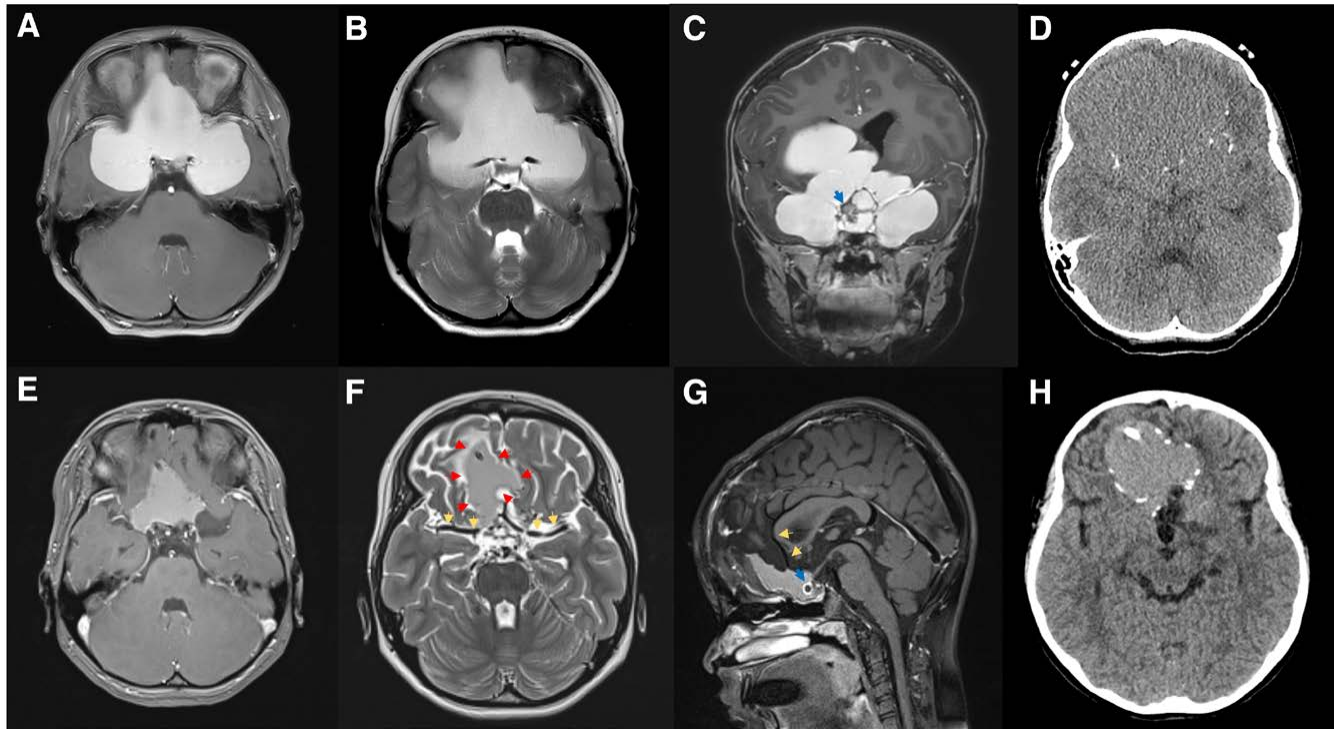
The radiologic findings of cystic craniopharyngioma following long-term cystic aspirations via the Ommaya reservoir. Preoperative brain magnetic resonance imaging (MRI) revealed a 10 cm-sized cystic mass involving both the anterior and middle cranial fossae. The cyst shows hyperintensity signals on (A) T1-weighted MRI and (B) T2-weighted MRI. (C) On contrast-enhanced T1-weighted MRI, the cyst, which was not enhanced by gadolinium, encased both optic nerves, the anterior cerebral artery (ACA), and the middle cerebral artery (MCA), and was accompanied by a solid mass (blue arrow) in the suprasellar area, suggesting a craniopharyngioma rather than a neurenteric cyst. (D) Brain computed tomography (CT) shows fine calcification along the cyst wall. Following long-term cystic aspirations through the Ommaya reservoir, the cyst shrank to 4.3 cm in size on (E) T1-weighted MRI. The tumor–pial plane (red arrows) became more distinct, and both MCAs were separated from the cyst (yellow arrows) on (F) T2-weighted MRI. The cyst was also separated from both ACAs (yellow arrows) and accompanied by a solid mass (blue arrow) on (G) contrast-enhanced T1-weighted MRI. (H) Brain CT, performed after cyst aspiration, shows more fine calcification along the cyst wall compared to the previous scan. ACA = anterior cerebral artery, CT = computed tomography, MCA = middle cerebral artery, MRI = magnetic resonance imaging.
